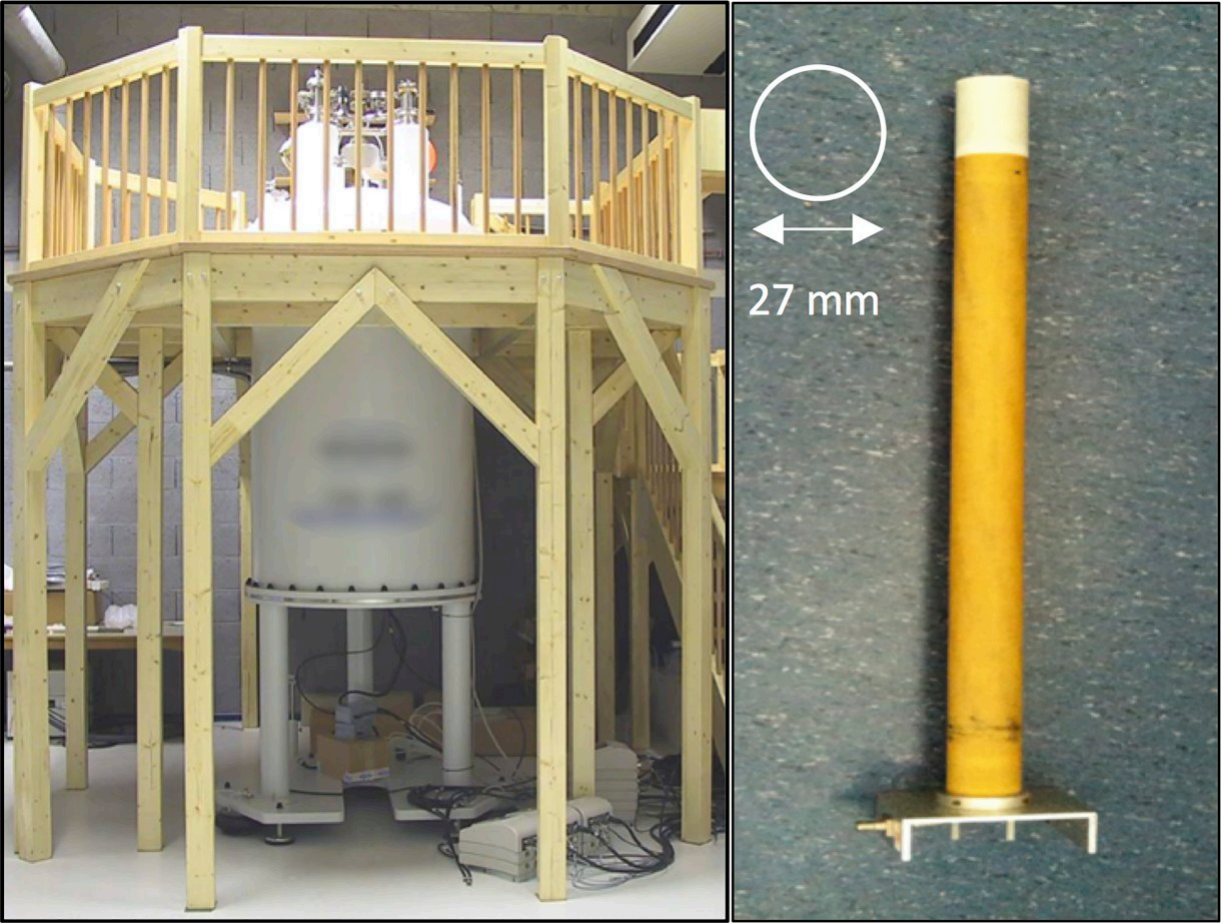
MRI hardware. Ultra highfield Bruker 17.6 Tesla MRI at Würzburg (left panel). Birdcage resonator with an inner diameter of 27 mm (right panel).
